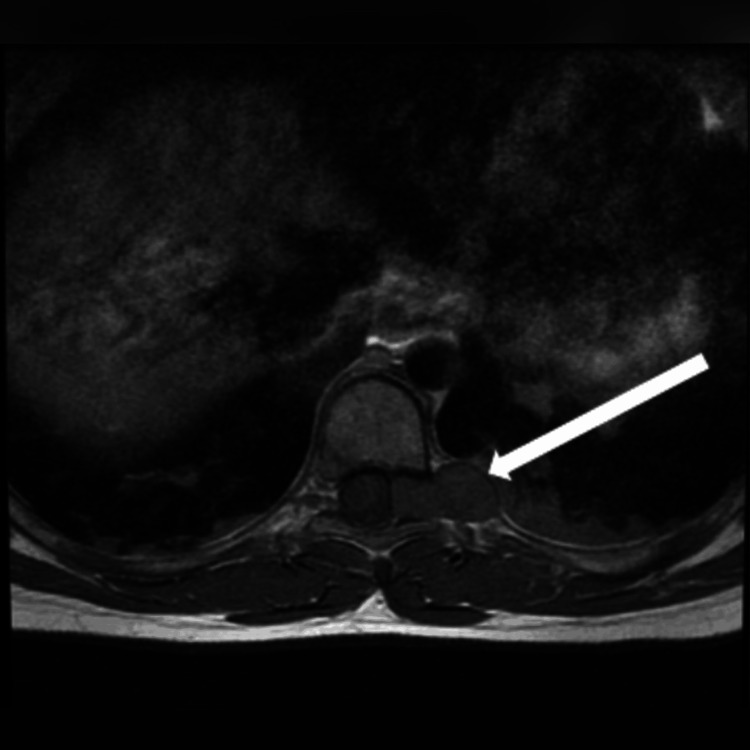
T1-weighted axial MRI showing a thoracic spinal schwannoma (arrow).
